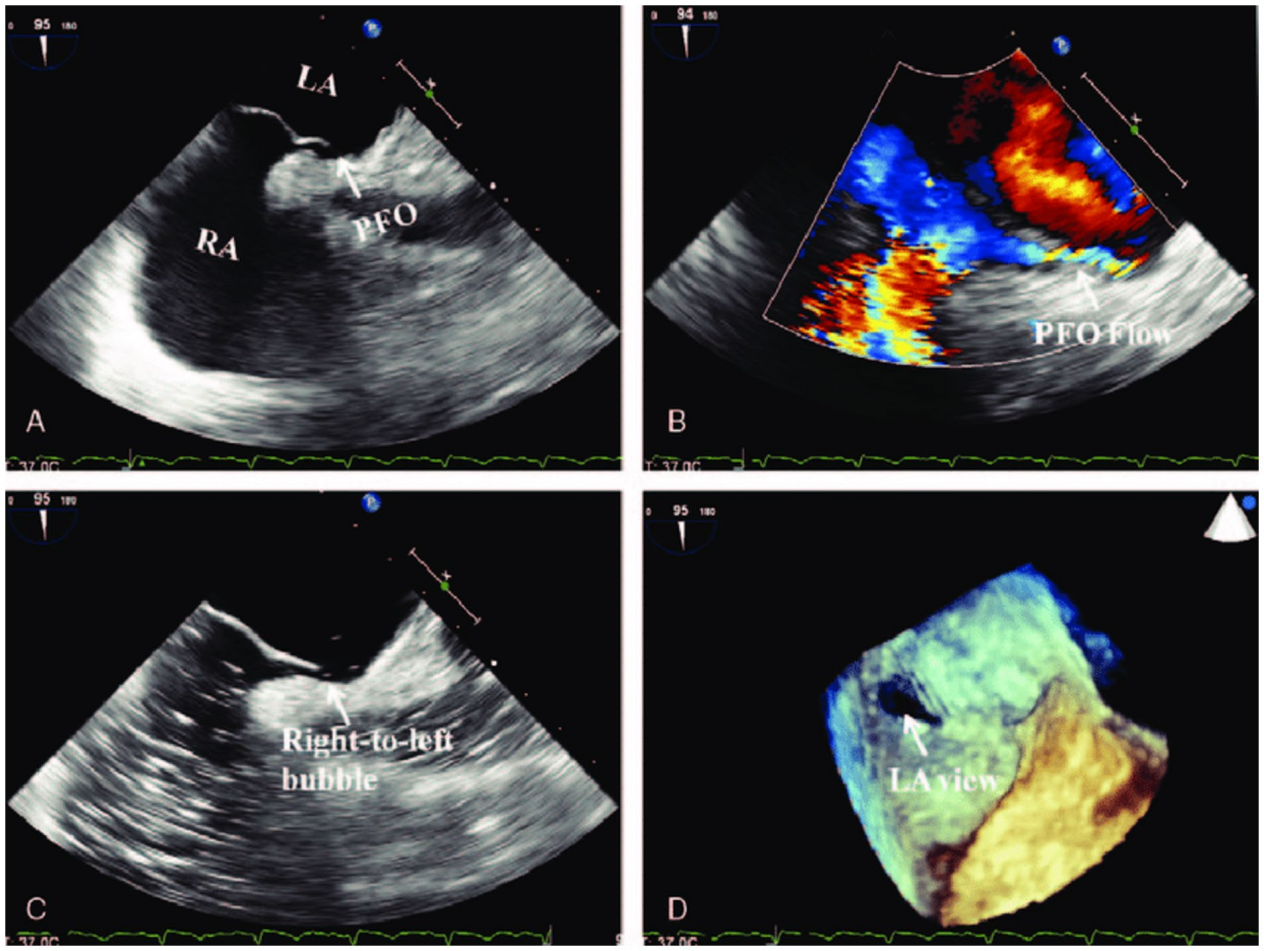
“Transesophageal echocardiography (TEE) confirming the PFO (arrow) (43). (A) The two-dimensional TEE in the biatrial view detected a separation between the primum and secundum atrial septum. (B) Color Doppler demonstrated bilateral but mainly right-to-left flow by decreasing the color gain and wall filtration. (C) Contrast TEE revealed right-to-left shunt after the contrast agent (6 mL of 1% injection vitamin B6 and equal volume of 5% sodium bicarbonate solution) was administered through the dorsal vein of right hand. (D) Real-time three-dimensional TEE confirmed the PFO. LA, left atrium; PFO, patent foramen ovale; RA, right atrium” by (Source Publication) is licensed under the Creative Commons Attribution-ShareAlike 4.0 International License.
